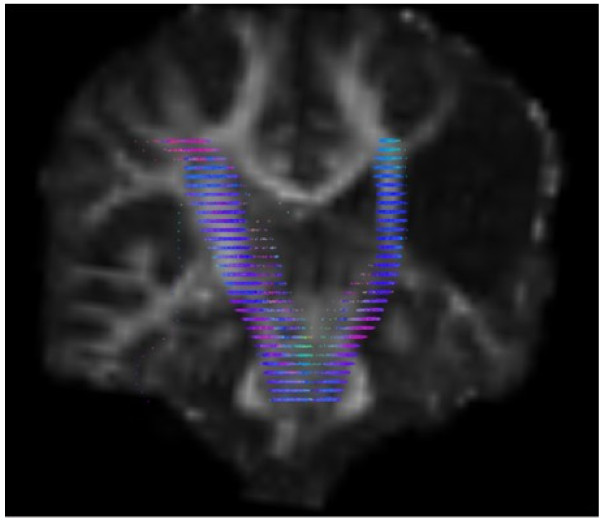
DWI Tractography Findings. Fractional anisotropy values were calculated at 2 mm intervals, resampled from the pons to the centrum semiovale. (R side of brain appears on L side of image).
